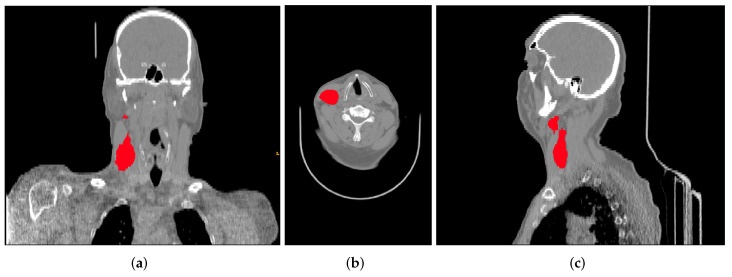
Example of a CT scan from the data collection with the corresponding segmentation of the gross tumour volume, shown in all three planes: (a) coronal, (b) axial, and (c) sagittal.
